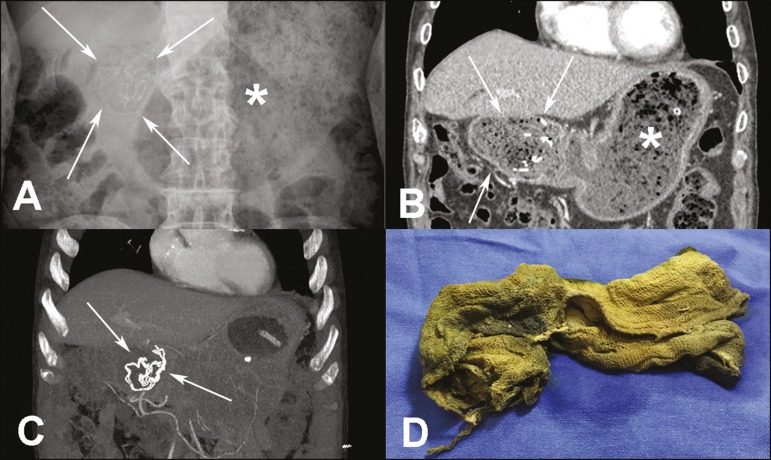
A: Conventional X-ray of the abdomen (with the patient in supine
